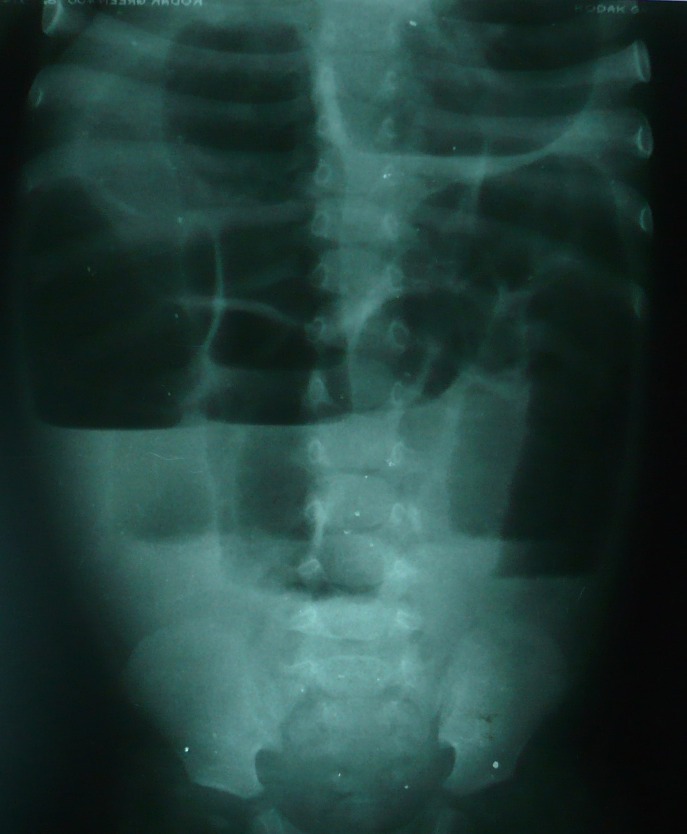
Figure 2: Erect radiograph showing multiple air fluid levels.
